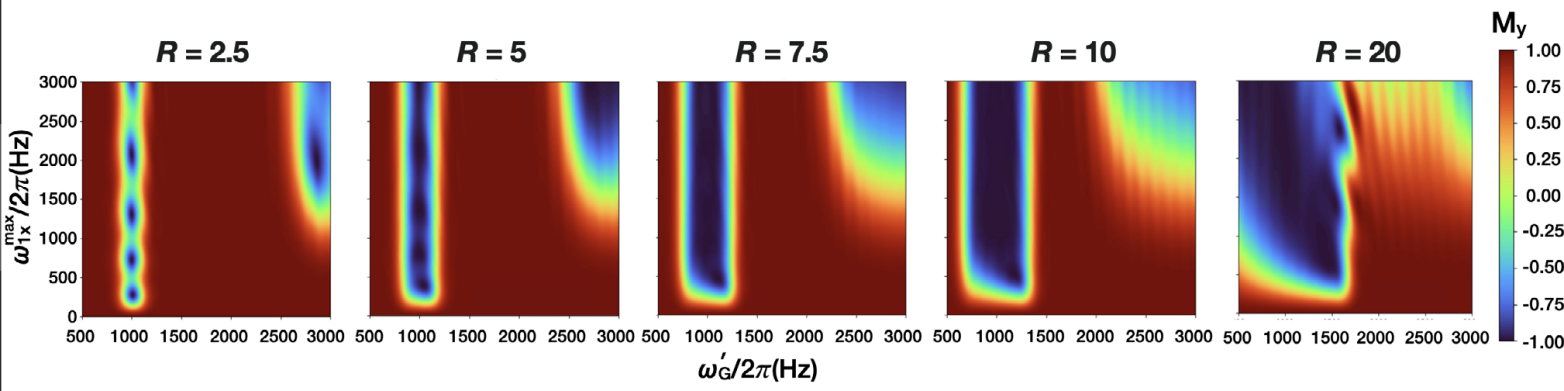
Slice inversion as a function of increasing $\omega_{1x}^{\max}/2\pi$ and increasing *R* value (time‐bandwidth product) for an AM_HS1_ pulse that is centered at $\omega_{1y}^{\prime }\left(r_{\text{center}}\right)/2\pi =1000\;\mathrm{Hz}$ and experiences a gradient of 500–3000 Hz, closer to the maximum RF amplitudes achievable with a head coil on a clinical MRI scanner.
